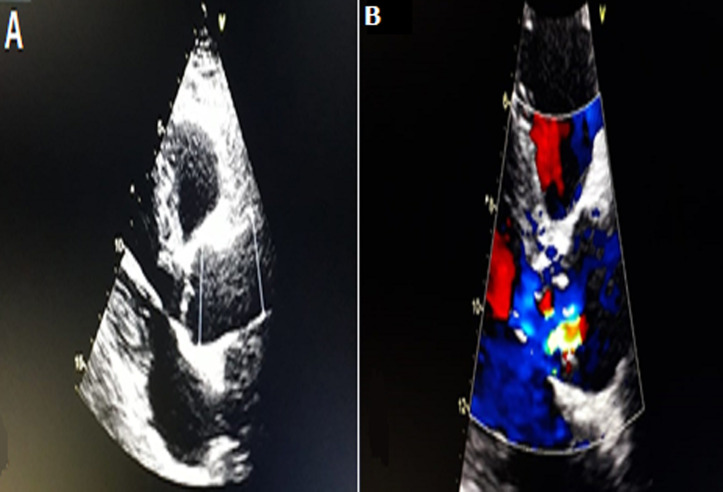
postoperative transthoracic echocardiography: (A) a parasternal long-axis view showing tube graft replacing the ascending aorta; (B) mild valvular AR in color doppler study
